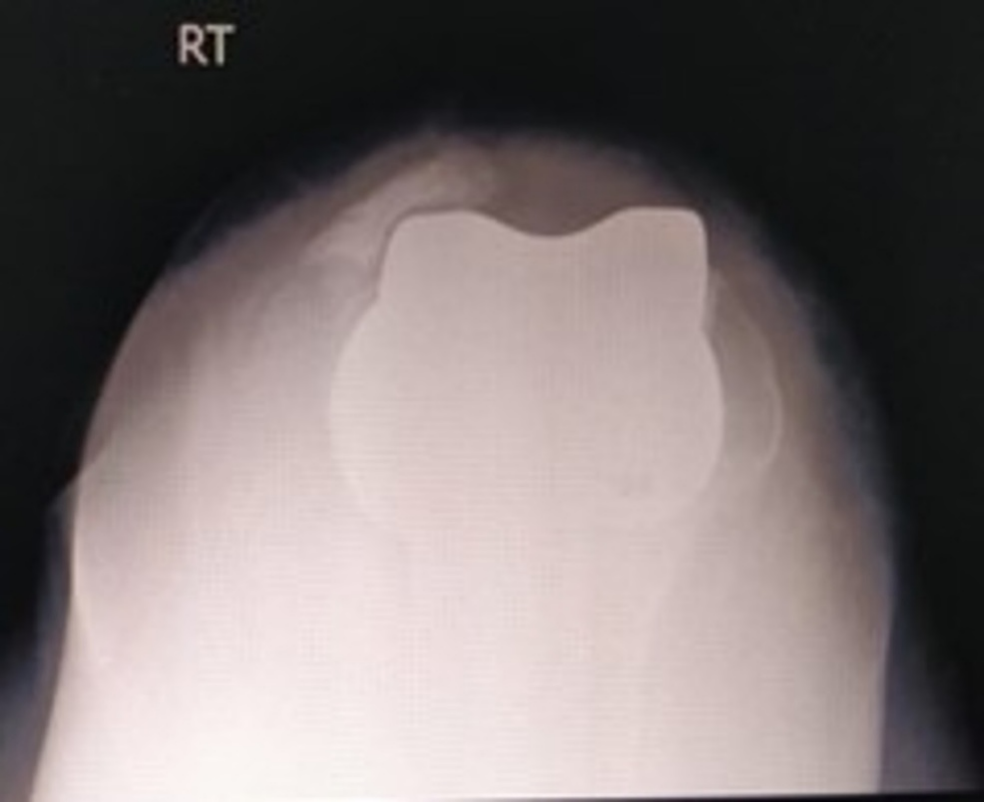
X-ray of the knee post total knee replacement showing the patella.
